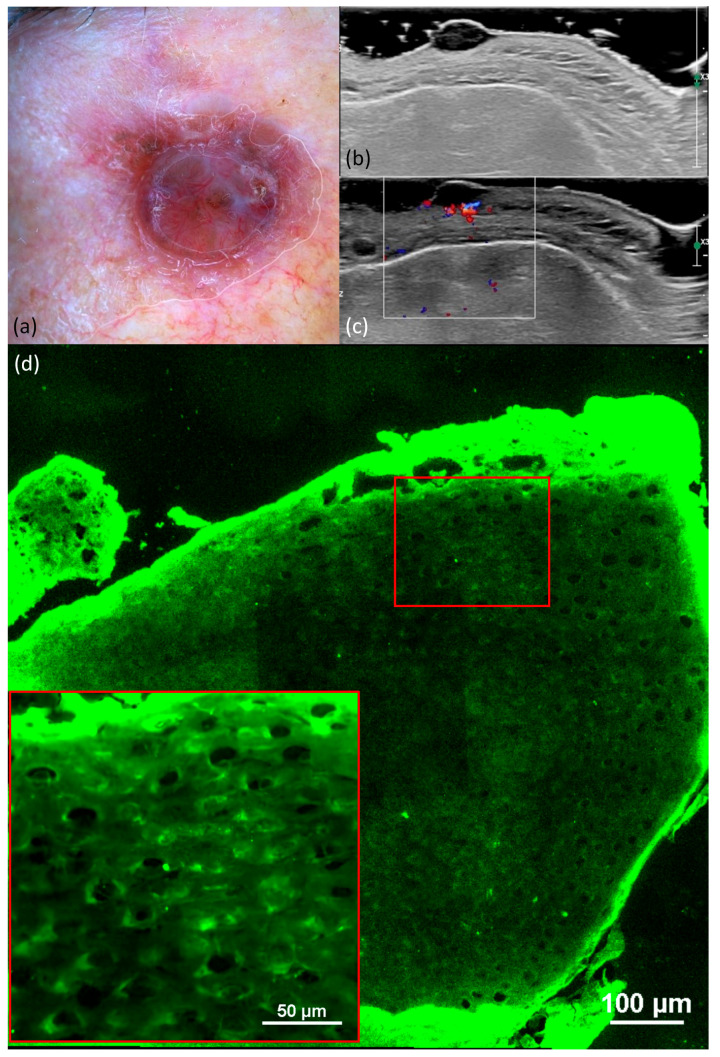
Nodular, adenoid BCC. Dermoscopy shows arborized vessels, blue-grey ovoid nests, and shiny white-red structureless areas (a). US shows a hypoechoic, oval, well-defined lesion that reaches the level of the reticular dermis and that contains hyperechoic points (b). Doppler mode shows an increase in tumor and peripheral vascularity (c). FCM shows well-defined tumor islands, palisading, and clefting (d).
